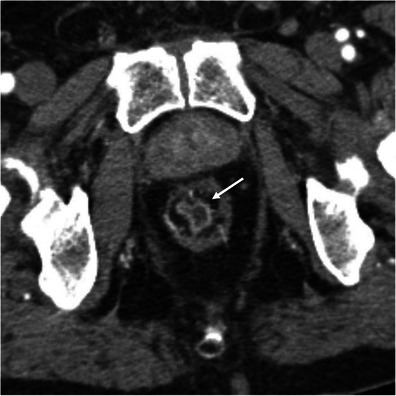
Fat halo sign in Crohn’s disease. Axial contrast-enhanced CT image shows concentric wall thickening of the rectum (*arrow*) with fatty appearance of the submucosa in a patient with a long history of Crohn’s disease
